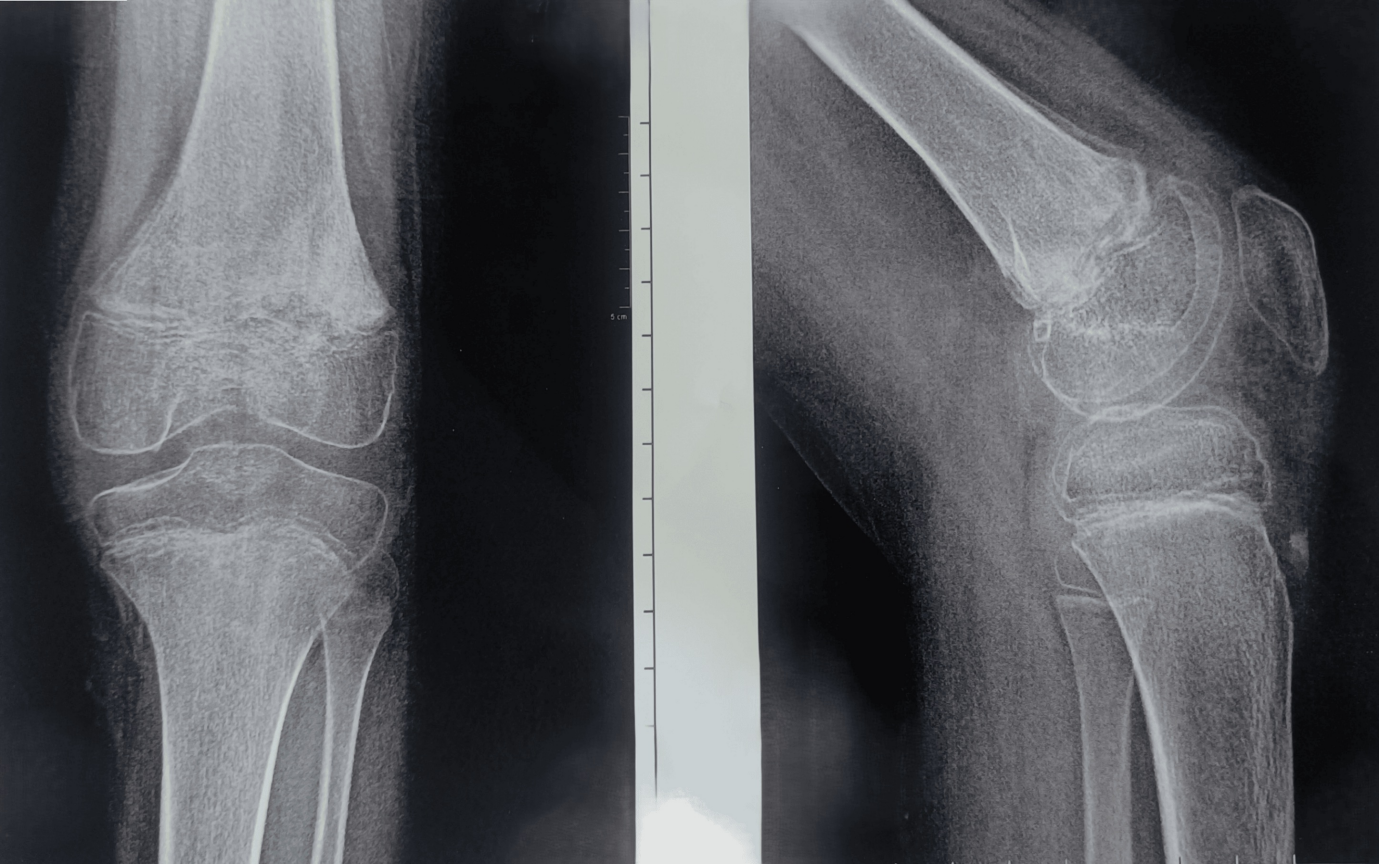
Post-operative X-ray after 15 weeks
